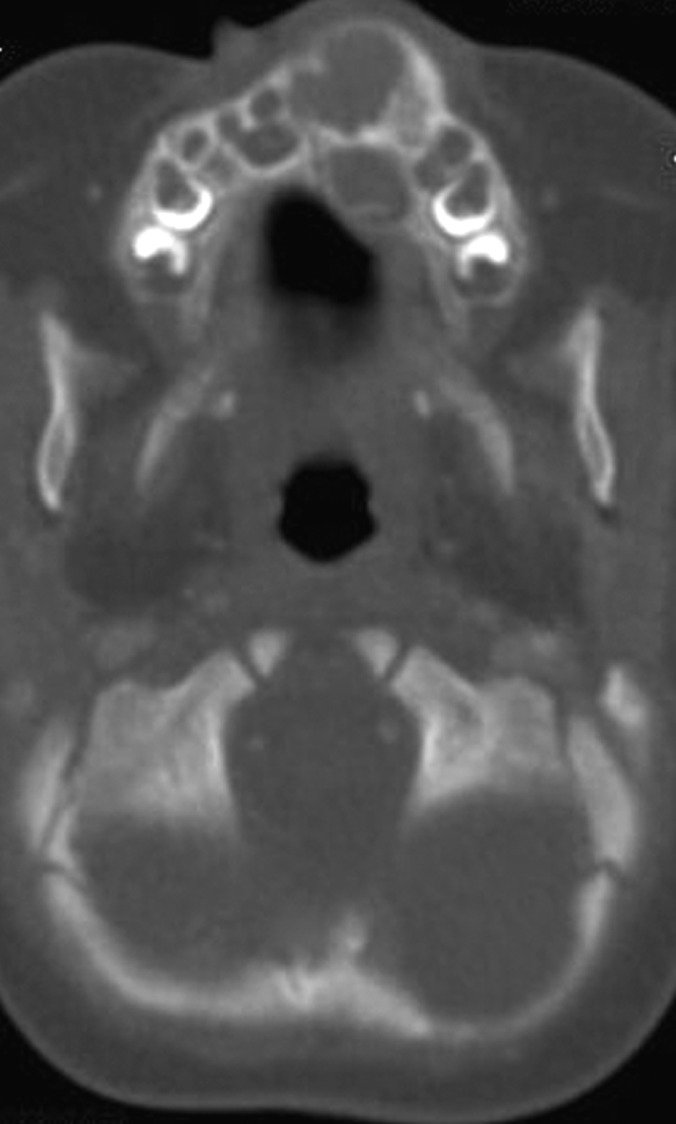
CT scan of the facial bones
